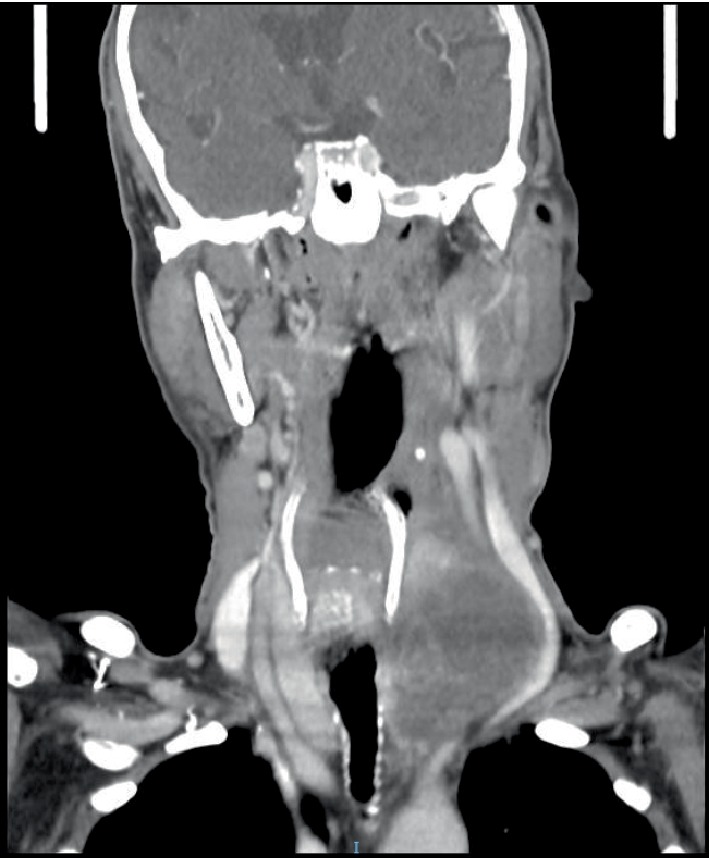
CT scan (coronal) showing gross deviation of the common carotid artery. The lack of fat plane suggests involvement of the carotid. The trachea is deviated but is not narrowed.
